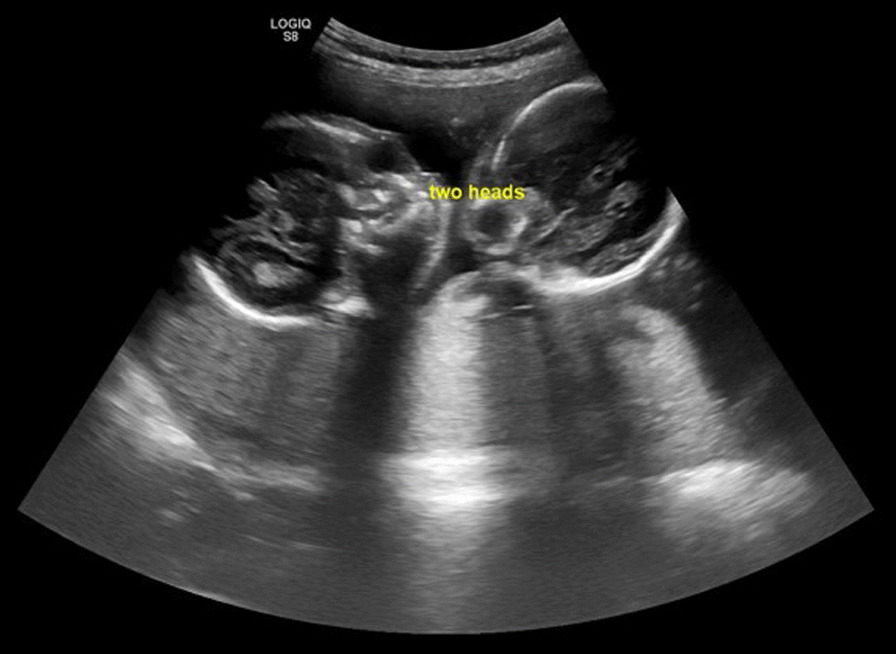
Axial ultrasound image showing two normally shaped fetal heads facing each other
